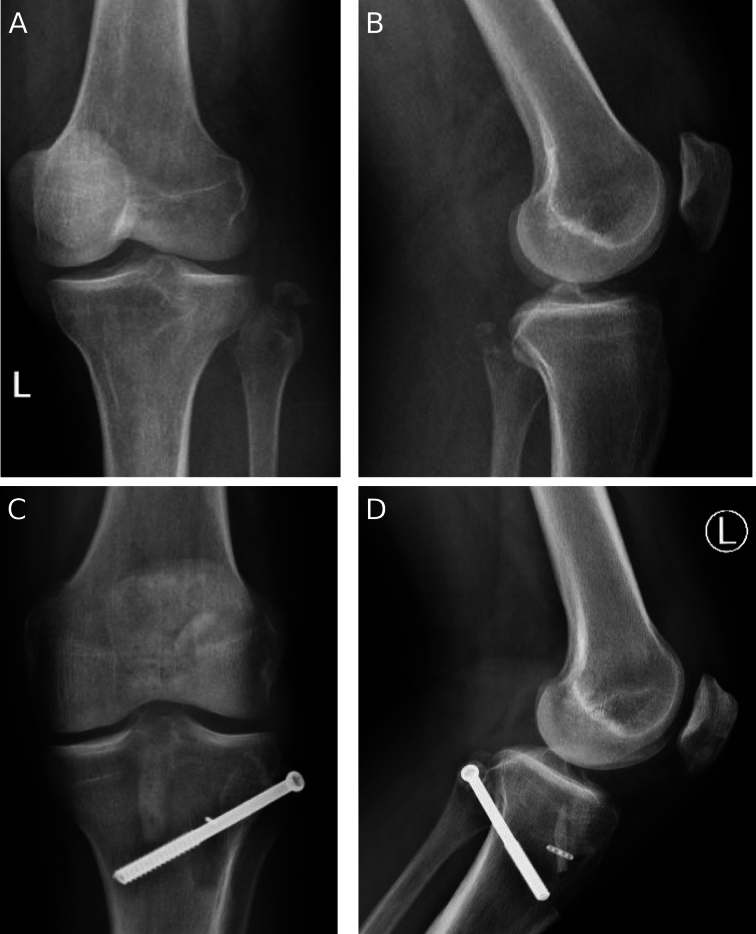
Pre-operative anteroposterior (a) and lateral radiographs (b) of a fibular avulsion fracture. Post-operative anteroposterior (c) and lateral radiographs (d) demonstrating the proximal tibiofibular screw fixation.
